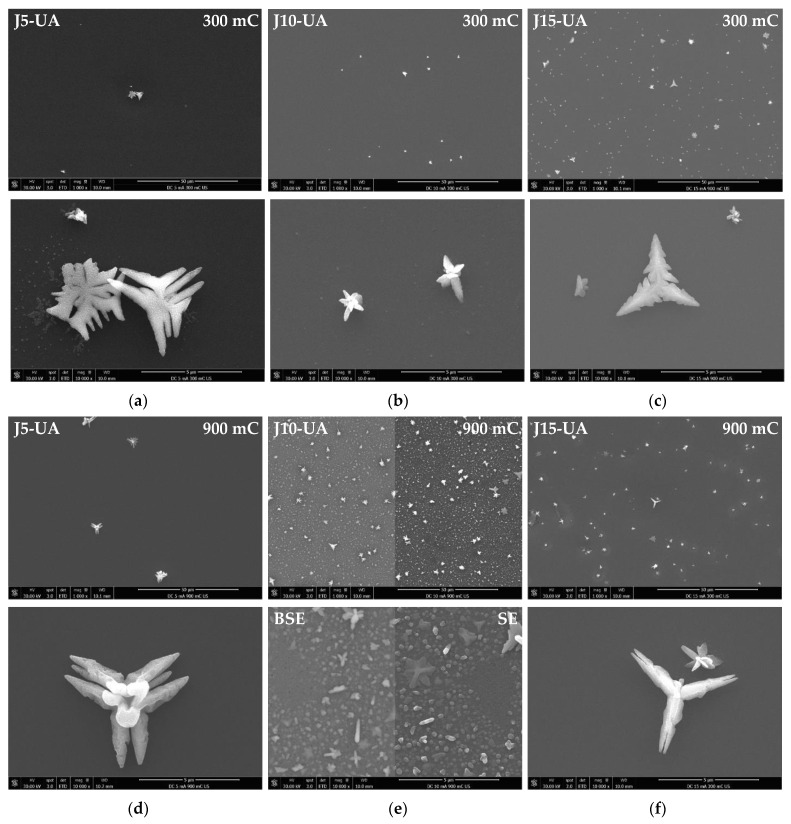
SEM images of the central regions of the as deposited Cu films with current densities of 5 (a), 10 (b), 15 mA·cm^−2^ (c) for 300 mC and at the same current densities in (d), (e), and (f), respectively, for 900 mC, under ultrasonic electrolyte agitation.
